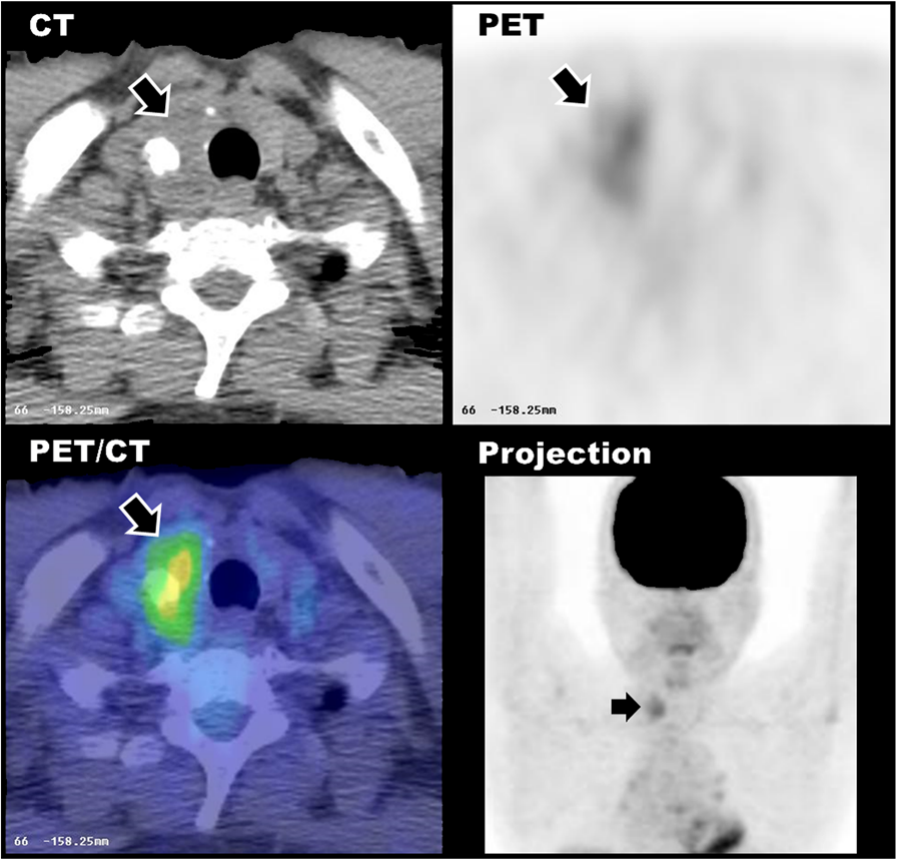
The second time FDG-PET/CT scan of the same patient with progressively elevated CEA levels up to 184 µg/L showed persistent focal FDG uptake in the right thyroid lobe (*arrow*), corresponding to the hypodense nodule and calcification shown on CT
